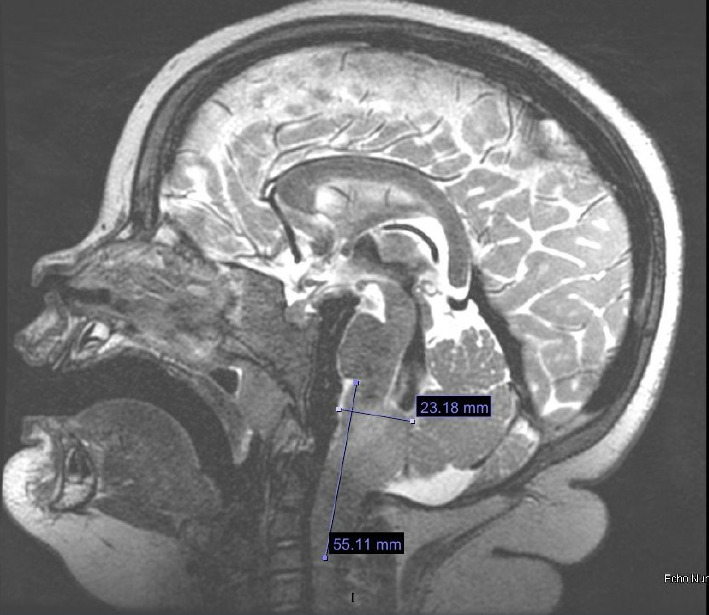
T2-weighted magnetic resonance imaging demonstrating the patient's medullary mass. Subsequent biopsy was consistent with ganglioglioma.
